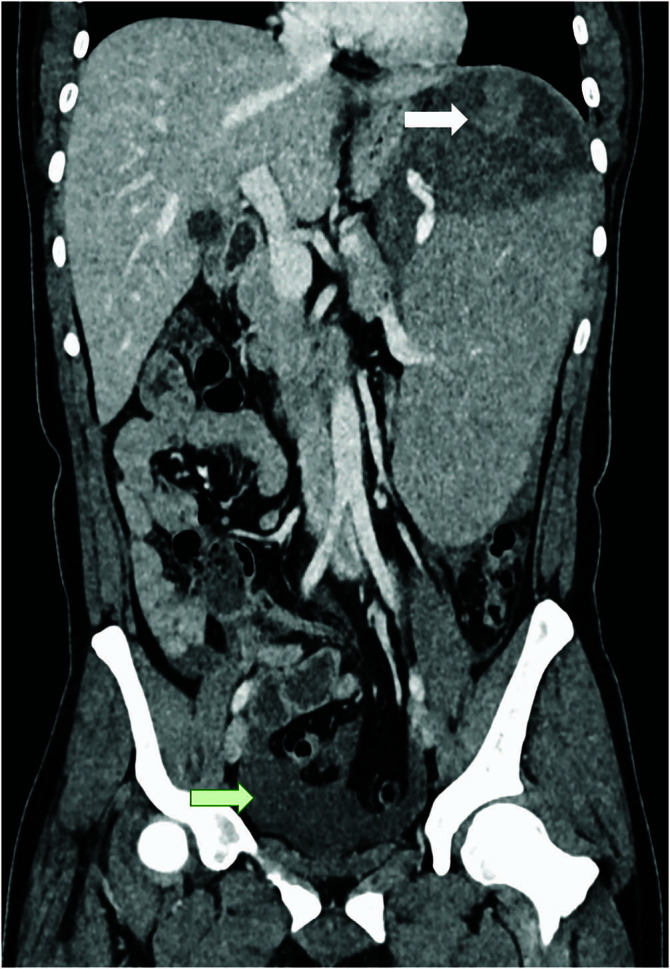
Coronal image of the CT scan of the abdomen with IV contrast in the venous phase showing a massively enlarged spleen measuring approximately 23 cm in craniocaudal dimension and an upper-pole splenic laceration (white arrow). Additionally, a mild-to-moderate hemoperitoneum can also be seen (green arrow).
